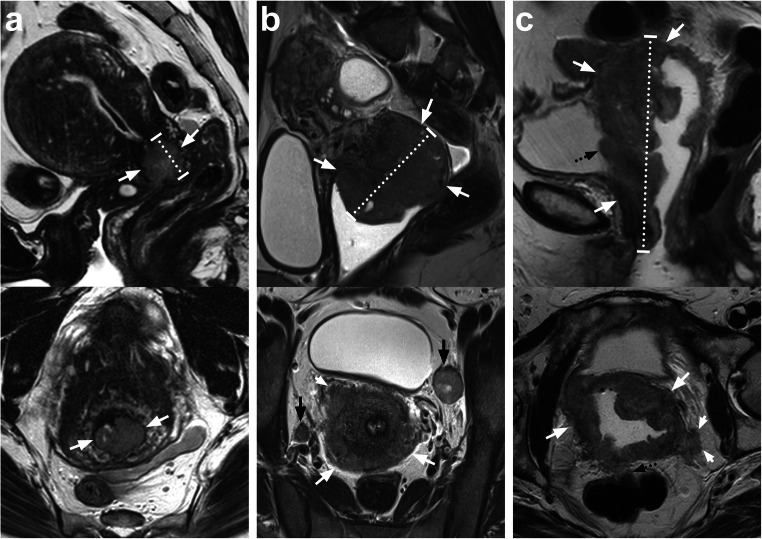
Cervical cancer depicted by sagittal (top) and axial oblique (bottom) T2-weighted MRI views in three patients. a A 40-year-old woman with a moderately large cervical cancer (white arrows) with a maximum tumor size of 2.4 cm (dotted line). The tumor is confined to the cervical stroma, and there are no enlarged lymph nodes (2018 FIGO IB2). The patient received primary surgical treatment (radical hysterectomy and salpingectomy) and had no signs of recurrence at 4 years post treatment. b A 23-year-old woman with a large cervical cancer (white arrows) with a maximum tumor size of 6.0 cm (dotted line). The tumor invades the parametrium (short white arrow), and bilateral enlarged pelvic lymph nodes are depicted (black arrows) (2018 FIGO IIIC1). The patient was treated with primary chemoradiation therapy and died from cervical cancer 2.5 years after primary diagnosis. c A 70-year-old woman with a large, irregular cervical cancer (white arrows) that extends to the uterine fundus and the lower third of the vagina. The maximum tumor size is 10.0 cm (dotted line) and tumor invades the parametrium (short white arrows) and both the bladder and rectum (black dotted arrows) (2018 FIGO IVA). The patient received primary chemoradiation therapy and died from cervical cancer 8 months after primary diagnosis. FIGO, International Federation of Gynecology and Obstetrics
